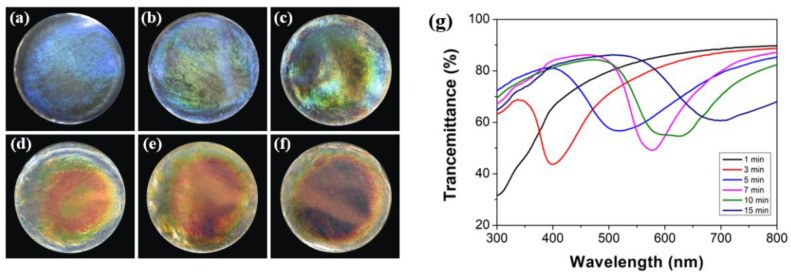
Photos of CNC films treated with different ultrasonic time (a) 1 min; (b) 3 min; (c) 5 min; (d) 7 min; (e) 10 min; (f) 15 min (d = 3.5 cm); (g) Transmission spectra of CNC films treated with different ultrasonic times.
